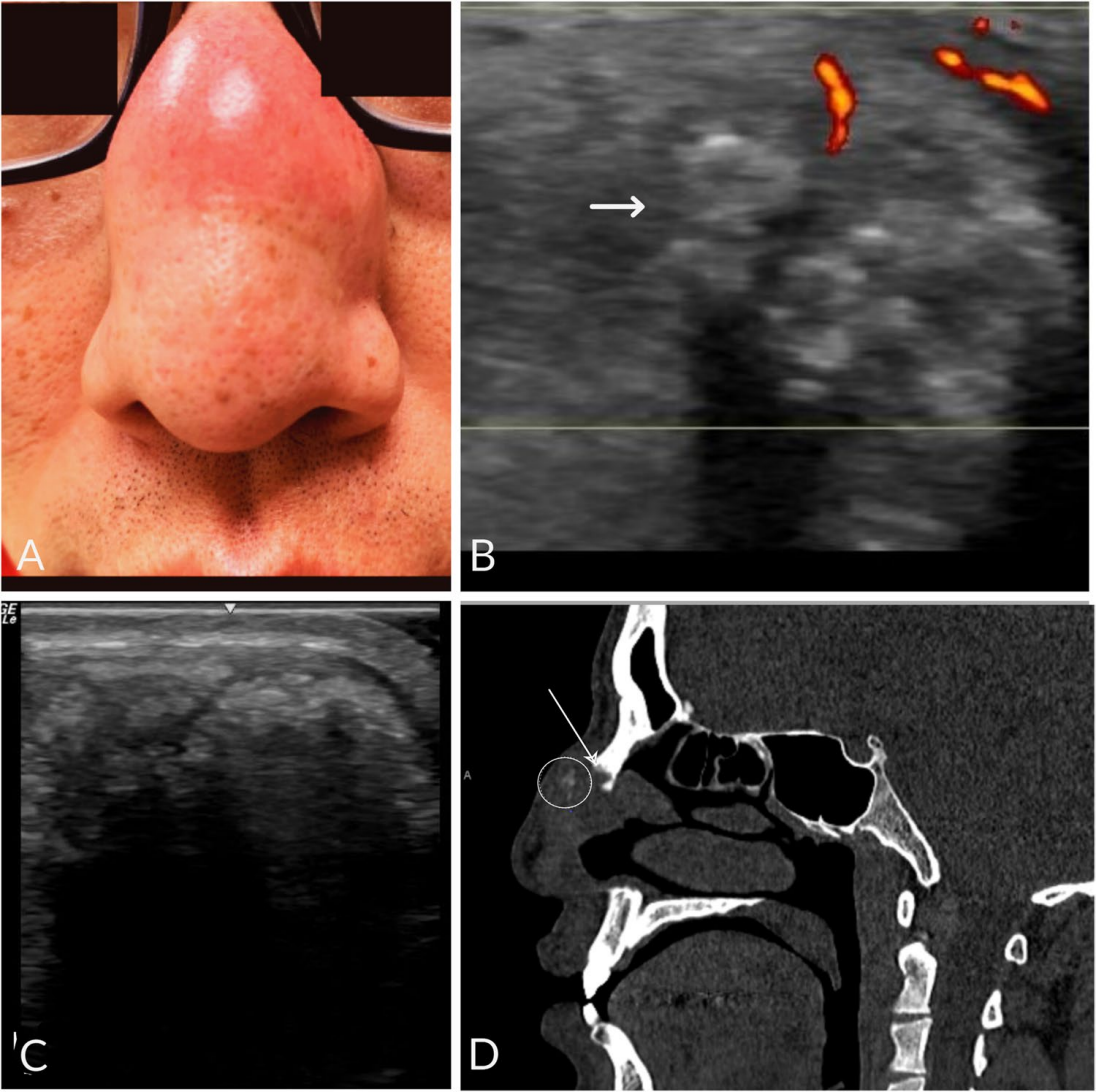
A Tender mass over nose. B Ultrasound of nasal bridge longitudinal view. C Transverse view—an area of inhomogeneous, hyperechoic aggregation with irregular margins, associated shadowing, and a surrounding hypoechoic rim compatible with a “wet clumps of sugar” appearance (white arrow). D Sinus CT without contrast shows a soft tissue mass with focal calcifications (circle) immediately adjacent to nasal bone erosion (arrow)
